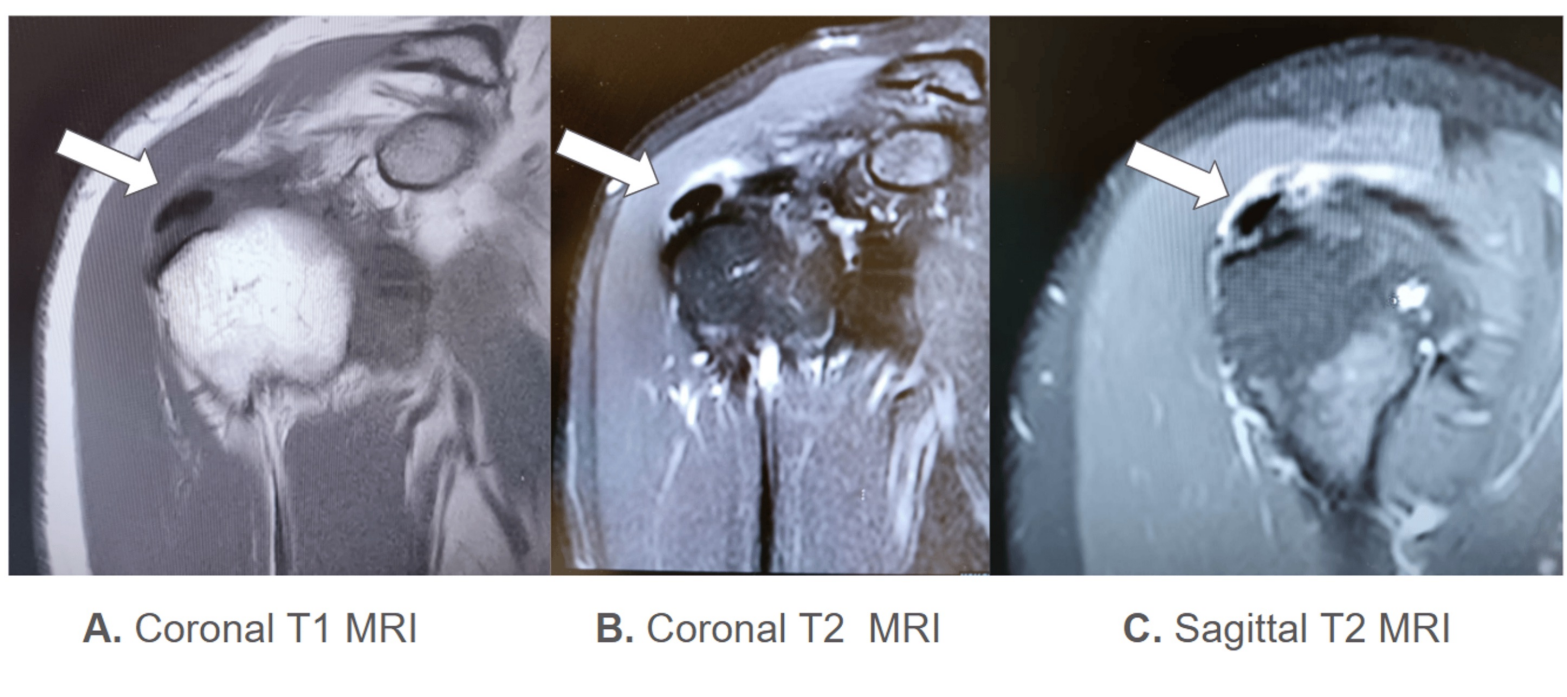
MRI showing calcification within supraspinatus tendon insertion
